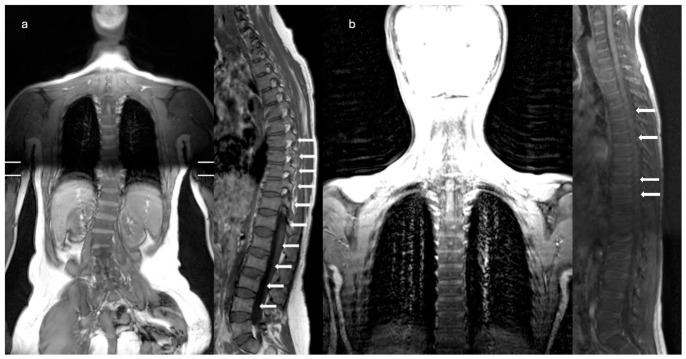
Comparative vertebral MRI scans of the mother (a) and son (b). Coronal T1/T2-weighted sequences highlight thoraco-lumbar scoliosis in the mother (a), versus no scoliosis in the son. Sagittal T1/T2-weighted sequences reveal chronic deformities with marrow changes in the mother as highlighted by the arrows (a), versus early biconcave fractures as highlighted by the arrows at T4, T6, T9, and T10 in the son, without canal compromise (b).
